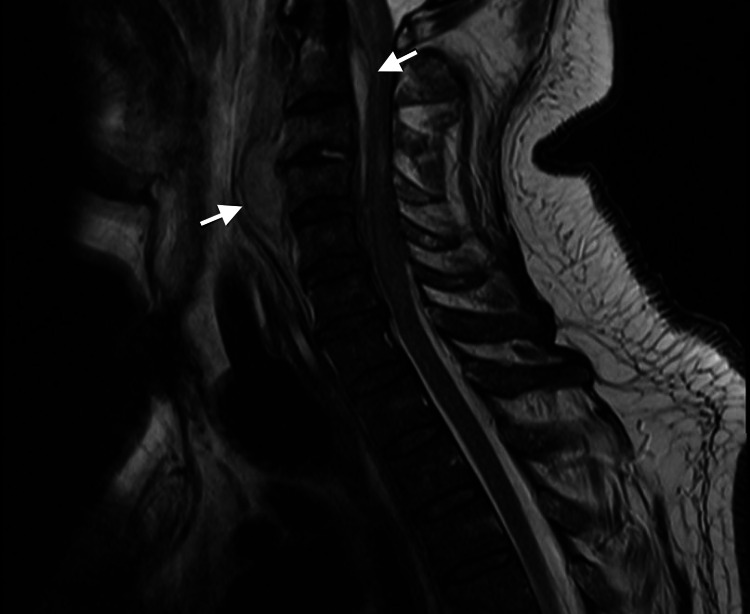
Sagittal T2 MRI revealing an anterior epidural abscess (C1-C4) compressing the spinal cord with high T2 signal and a prevertebral abscess (C3-C4).
